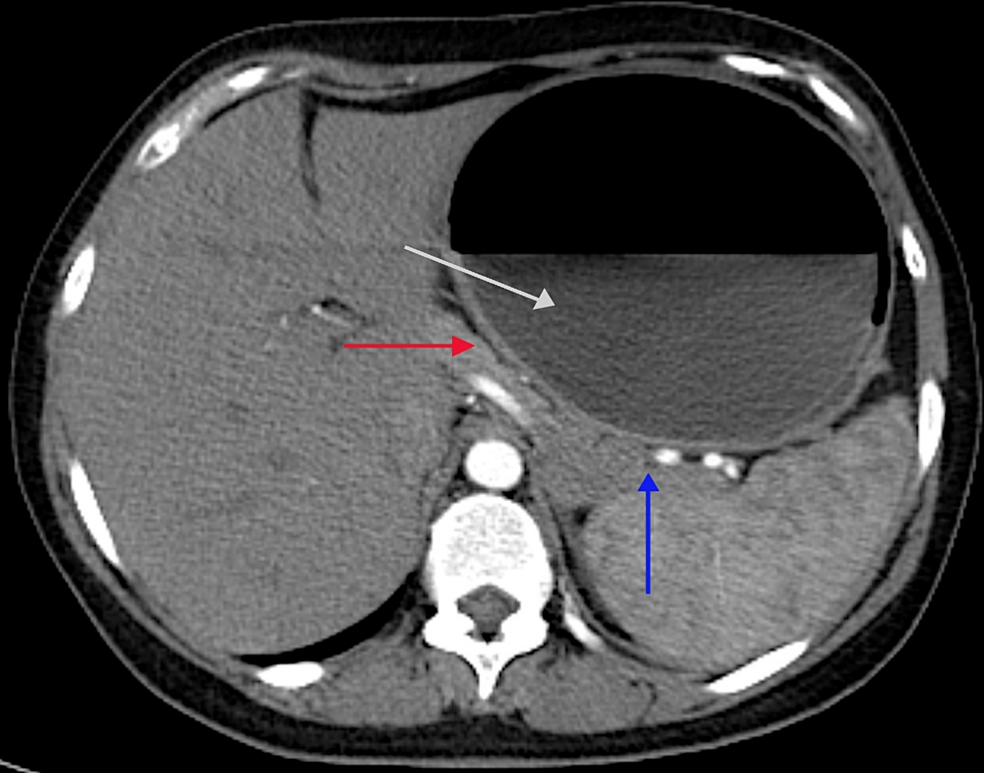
Computed tomography scan of the abdomen in the axial plane, showing the over-distended balloon half filled with fluid and containing air-fluid level (white arrow) compressing the pancreatic head (red arrow) with fluid and fat stranding surrounding the pancreatic tail (blue arrow).
